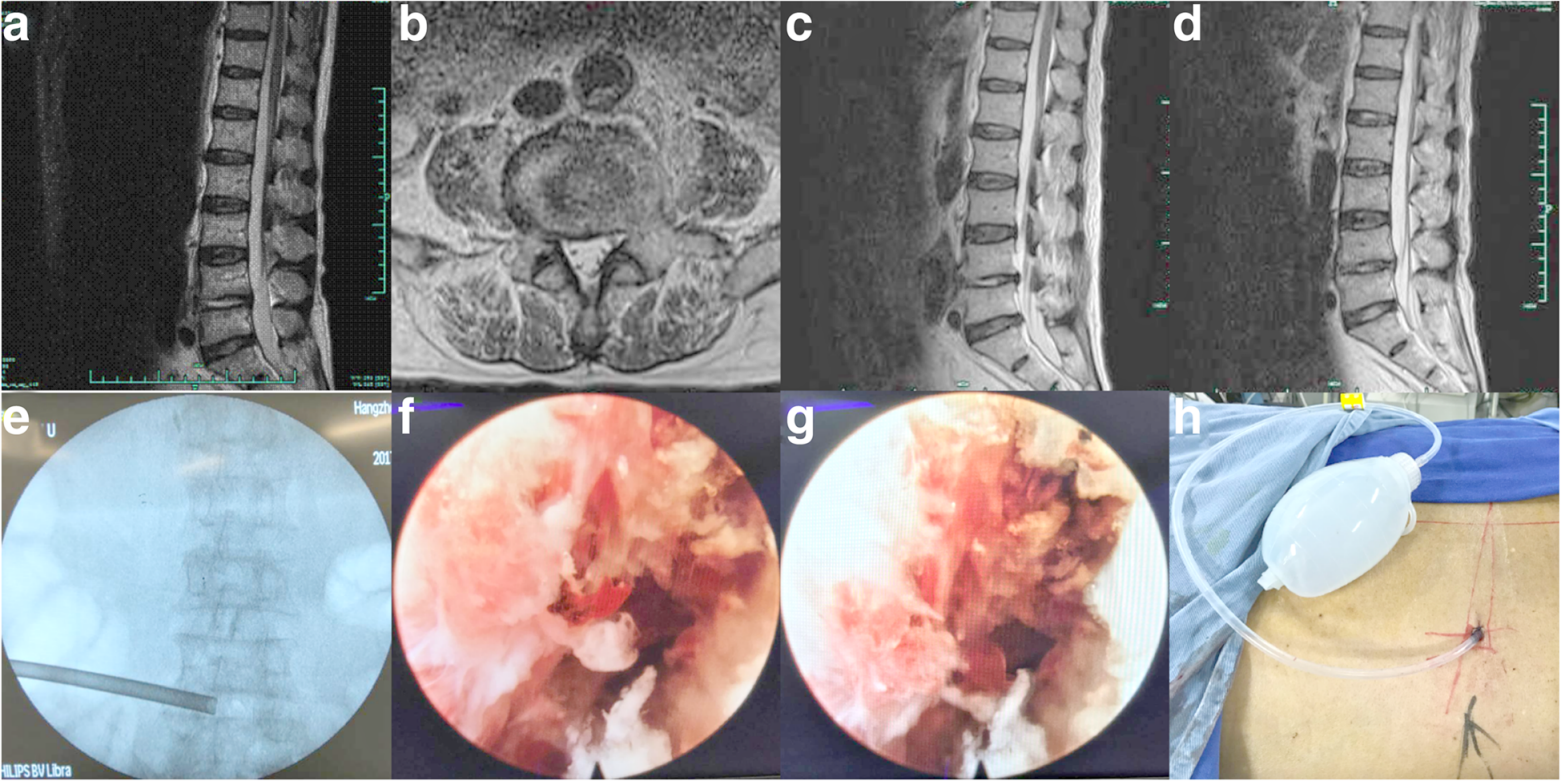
Typical case (case number 2). A 71-year-old man was diagnosed with having L5–S1 infectious spondylitis. A sagittal and axial T2-weighted MRI revealed L5–S1 infection with a paraspinal abscess (a, b). Unilateral percutaneous endoscopic debridement and drainage was performed (e, h). On endoscopic views, after the necrotic tissue and pus were discharged, the chapped disc was evident (f, g). Postoperative sagittal T2-weighted MRI at the 1 week (c) and 1 month (d) follow-ups demonstrated a decrease in the abnormally high signal in the L5/S1 intervertebral disc and a disappearance of the abscess.
